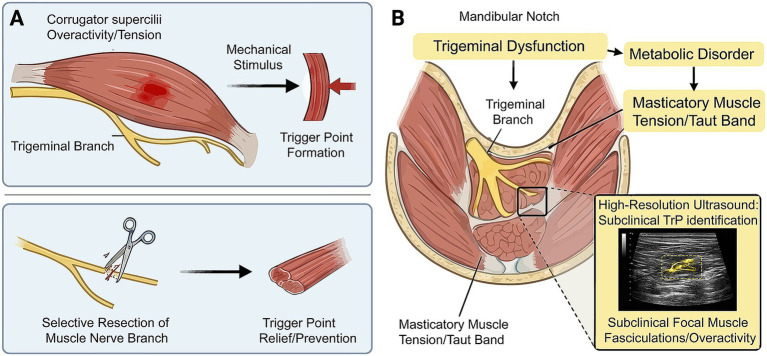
Two proposed muscle-nerve interaction pathways contributing to trigger zone formation. (A) Shows that *corrugator supercilii* overactivity imposes mechanical stress on the adjacent trigeminal branch, and selective nerve branch resection achieves sustained relief. (B) Shows that masticatory muscle tension produces subclinical fasciculations detectable by high-resolution ultrasound.
